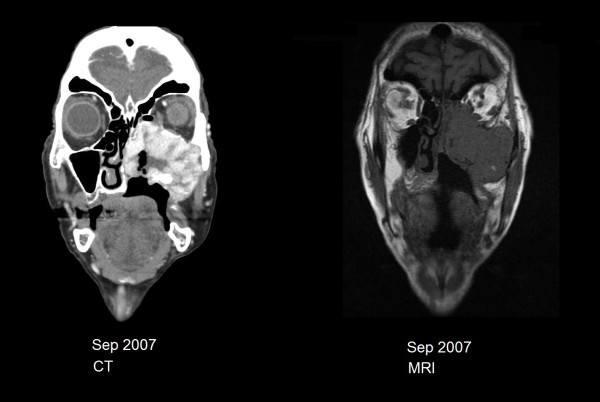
Coronal head and neck computed tomography (left) and magnetic resonance imaging (right) showing tumour size at presentation.
